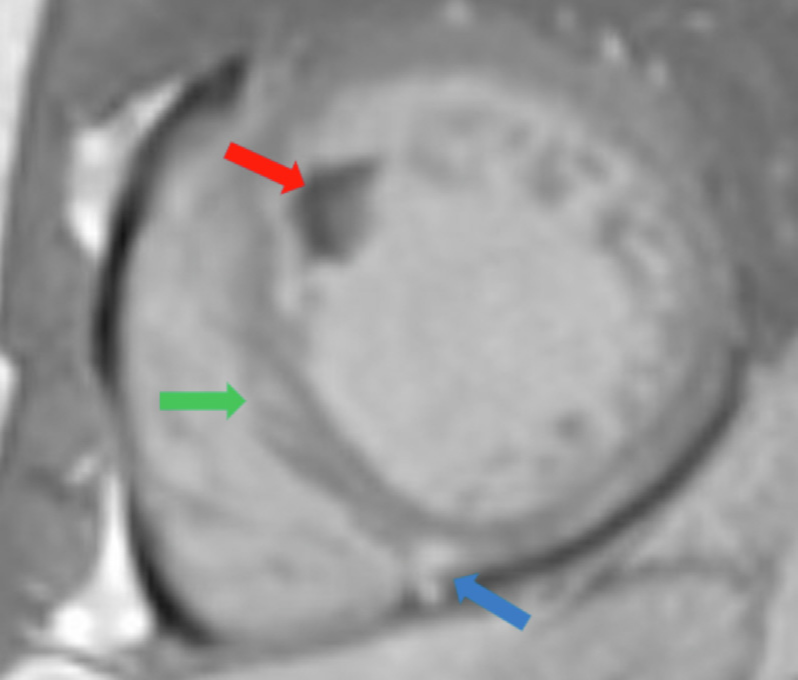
Thrombus on Cardiac MRI Apical thrombus (red arrow), with focal gadolinium uptake at the right ventricular insertion point (blue arrow), which suggests a dilated cardiomyopathy with some septal uptake (green arrow) noted on short-axis delayed phase-sensitive inversion recovery.
